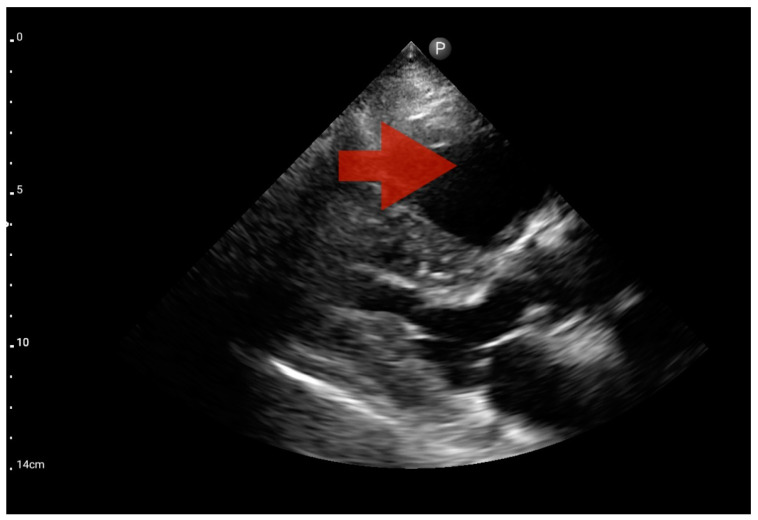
Right ventricle enlargement; position: parasternal long axis (FATE protocol). Sector probe; the right ventricle is marked with the red arrow, and a significant enlargement of the right ventricle is visible, with a shift of the interventricular septum towards the left ventricle, and an ultrasound picture suggesting pulmonary embolism (source: author’s material—DK).
